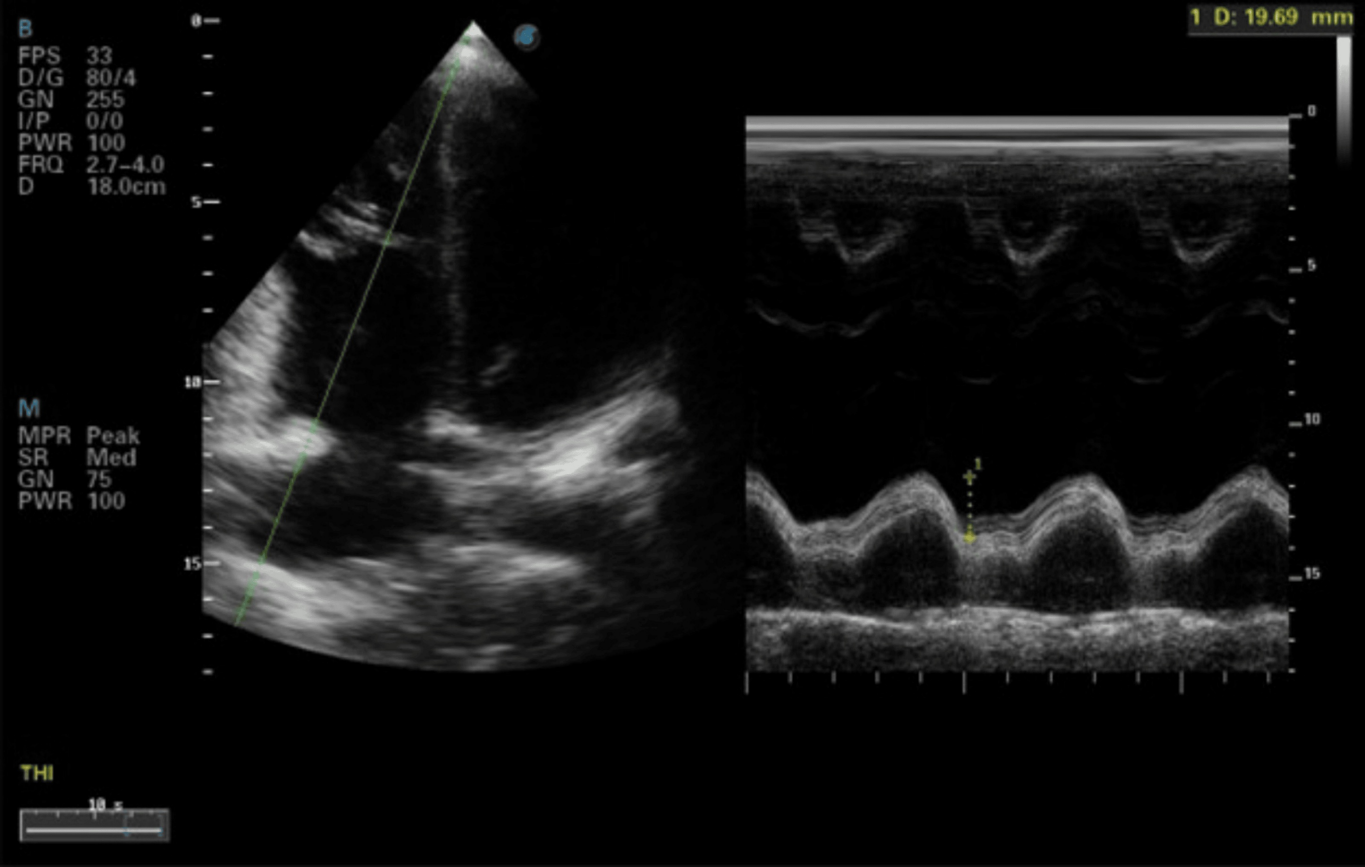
Echocardiography picture showing preserved RV systolic function with TAPSE of 19 mm RV, right ventricular; TAPSE, tricuspid annular plane systolic excursion
